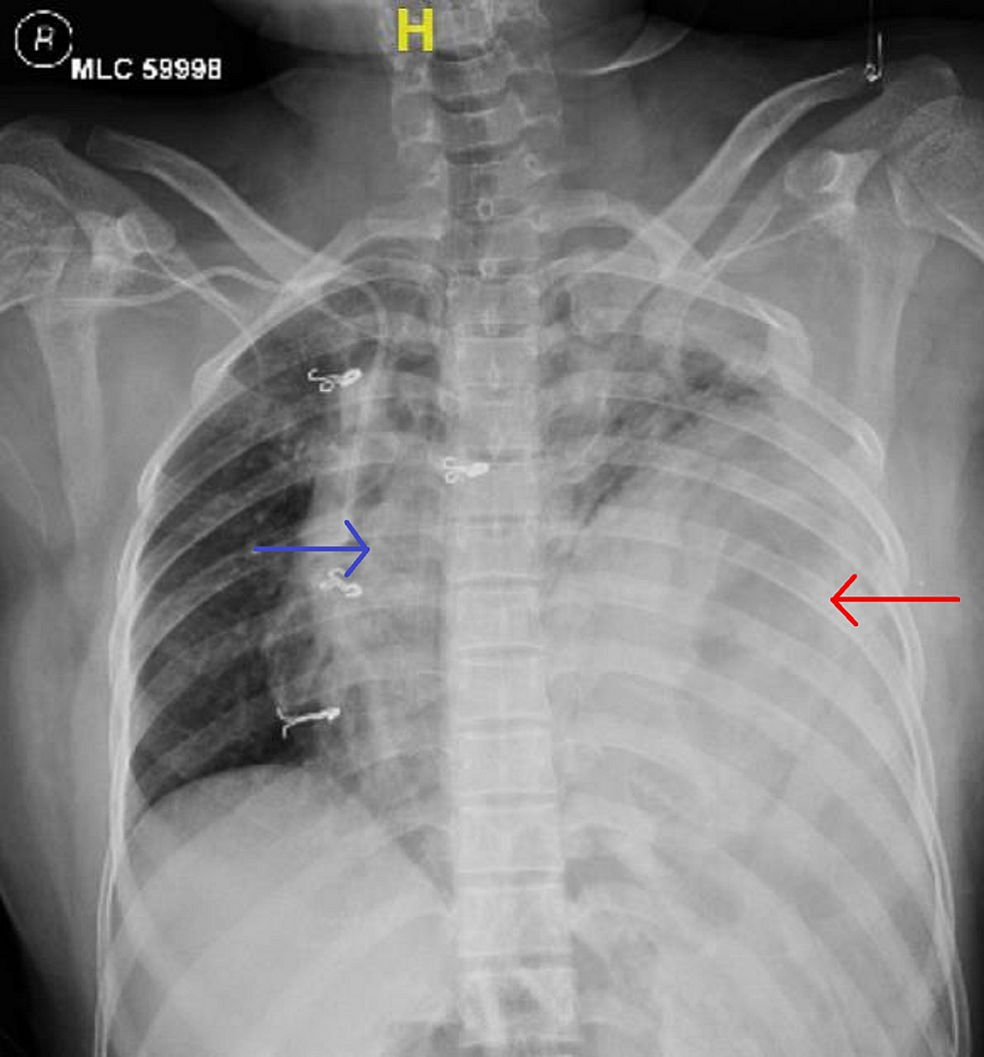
Chest X-ray A blue arrow shows the mediastinal shift and a red arrow shows the herniated bowel in the left hemithorax.
